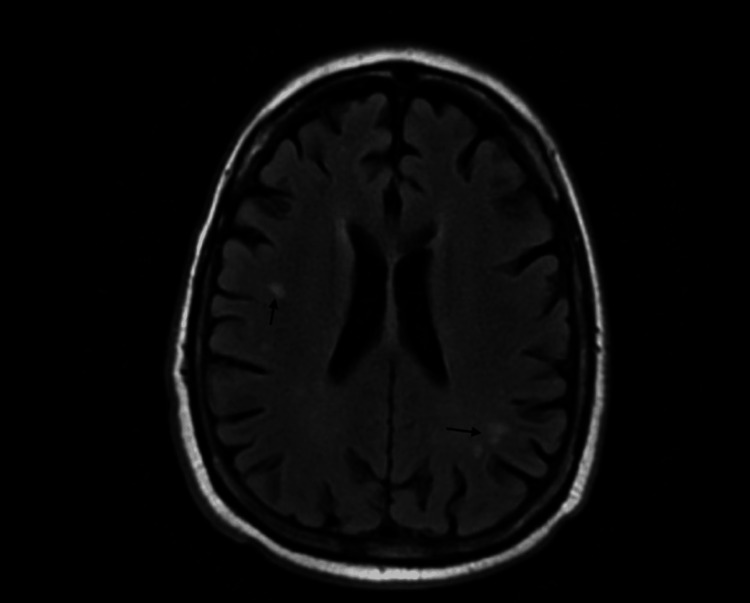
White matter changes in brain MRI MRI: magnetic resonance imaging
